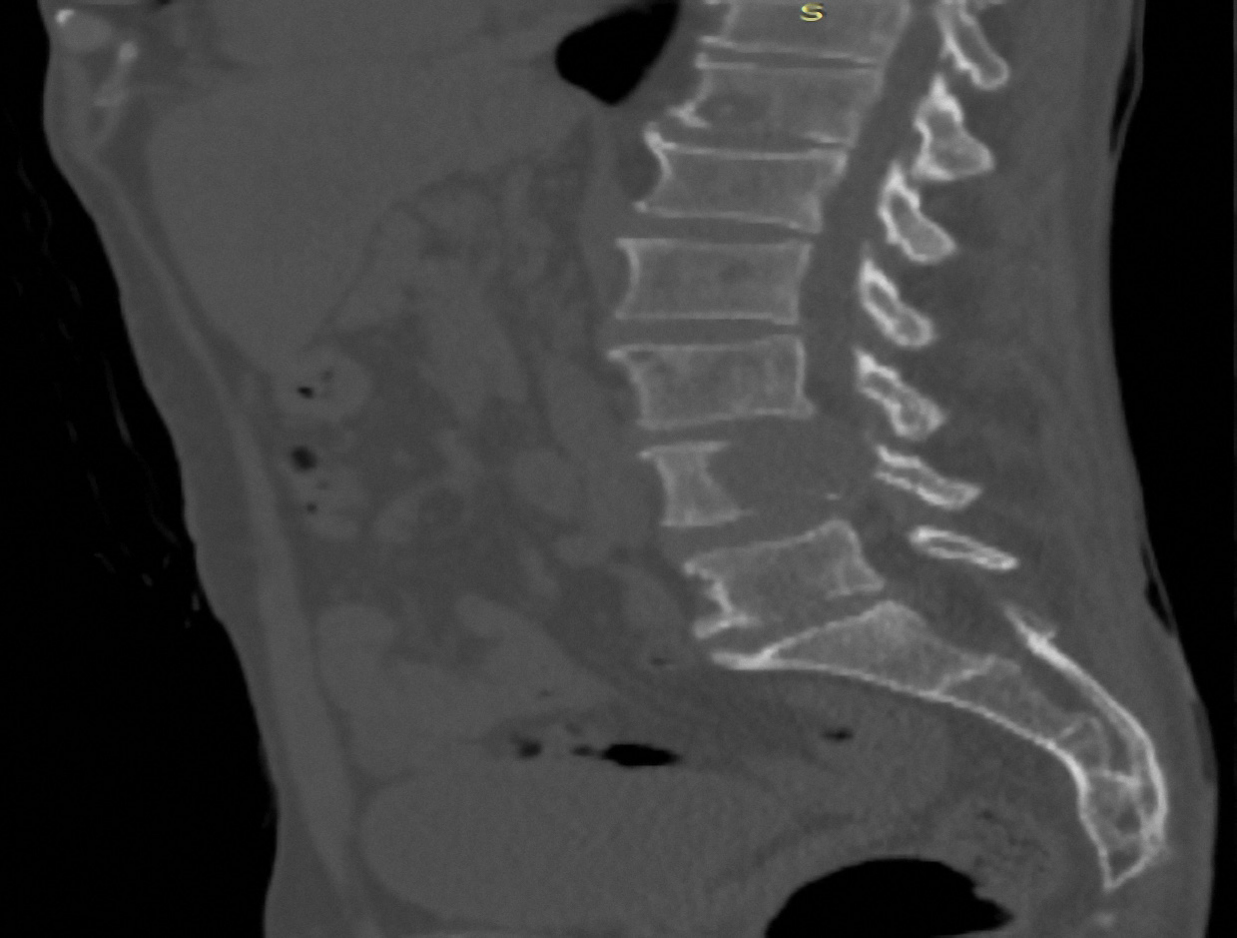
Thoracic-abdominal-pelvic (TAP) CT scan – bone window: osteolytic lesion in the L4 vertebral body with intracanalar extension and narrowing of a third of the vertebral canal.
